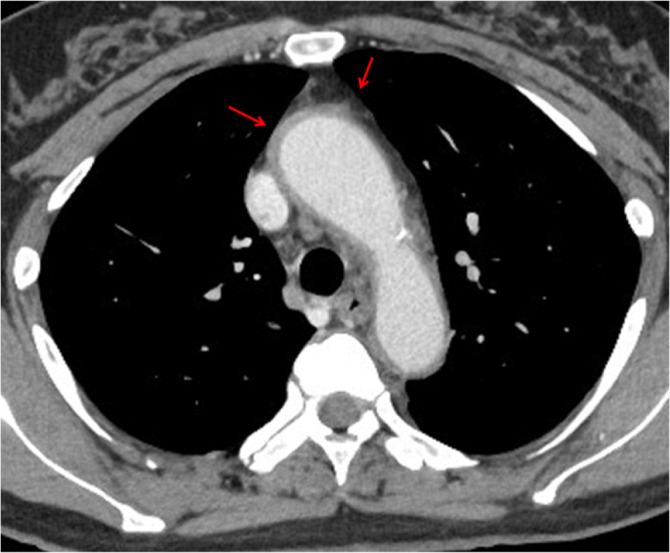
Contrast-enhanced computed tomography reveals thickening of the wall of the ascending aorta and aortic arch with fat stranding (red arrows).
